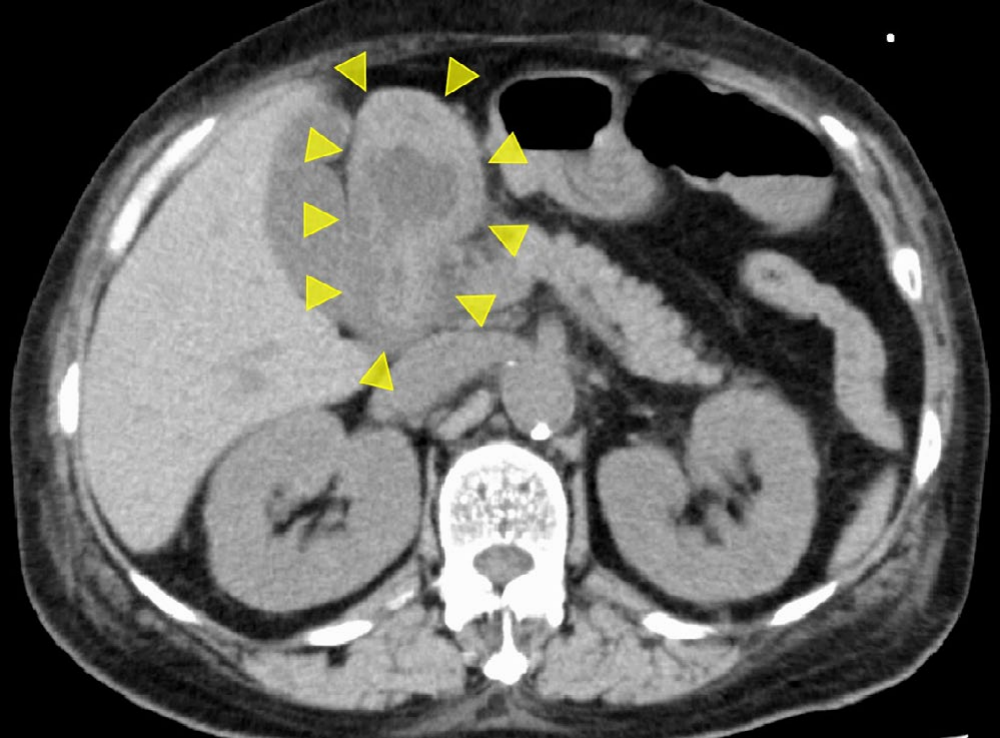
CT image. The duodenal wall is markedly thickened (arrowheads)
